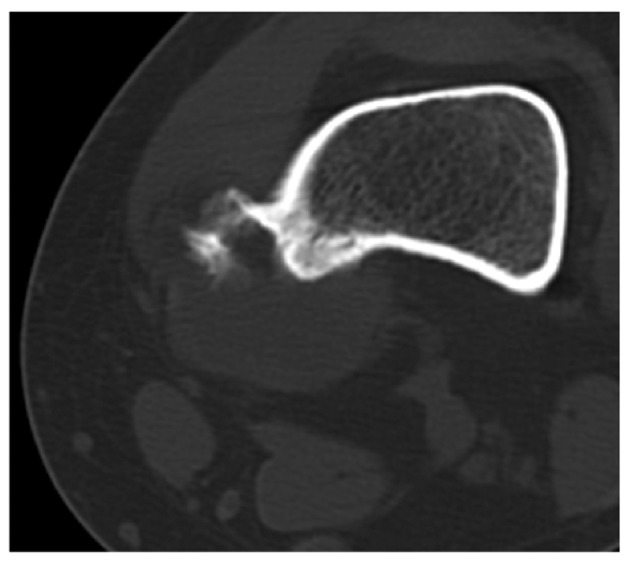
Axial computed tomography revealing that the tumor contained calcific densities characteristic of the external bone surface and associated with a thickened cortex, which indicates a periosteal chondrogenic tumor.
